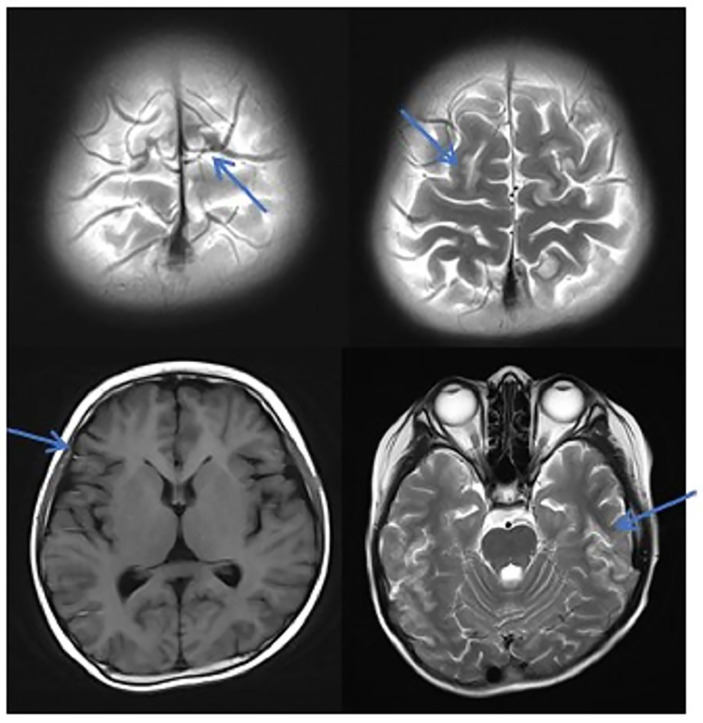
MRI in patients with TA-TMA. Patients with TA-TMA showed bilateral frontal, parietal, and occipital sulcus edges with a sheet-like low signal on the T1WI, a slightly high signal on the T2WI, a FLAIR high signal, unclear borders, a bilateral parietal dotted DWI high signal, and unclear borders.
